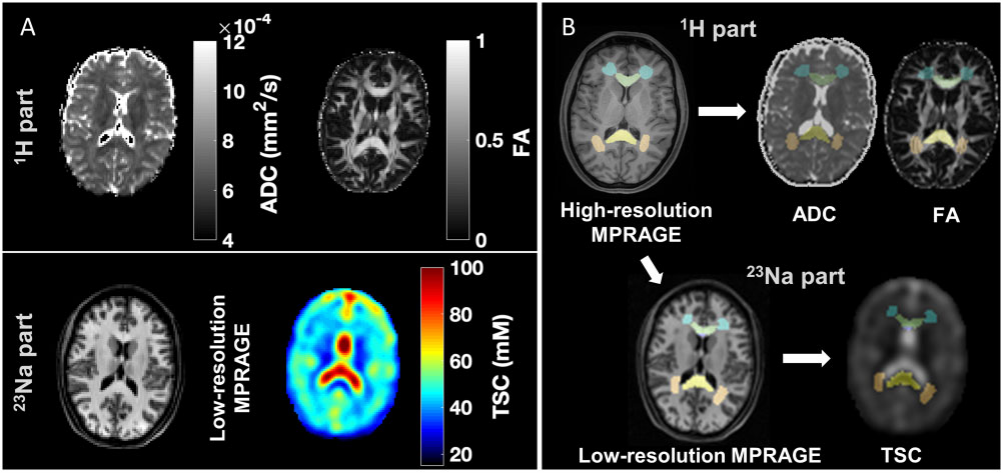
Example of DTI and sodium MRI, segmentation and registration. (A) The protocol consisted of two sessions, each done with a different RF coil. *Top*: during the ^1^H part, apparent diffusion coefficient (ADC) and fractional anisotropy (FA) maps were obtained from DTI. *Bottom*: For ^23^Na MRI, total sodium concentration maps (TSC) and low-resolution MPRAGE images for registration were acquired. (B) Regions of interest were obtained from high-resolution MPRAGE images and then registered to the TSC using the low-resolution MPRAGE images, and to the diffusion maps using the *b*_0_ image from the diffusion acquisition. Note the accuracy of the registration, e.g. for the genu and splenium of the corpus callosum, frontal and posterior WM (green, yellow, blue and pink, respectively).
